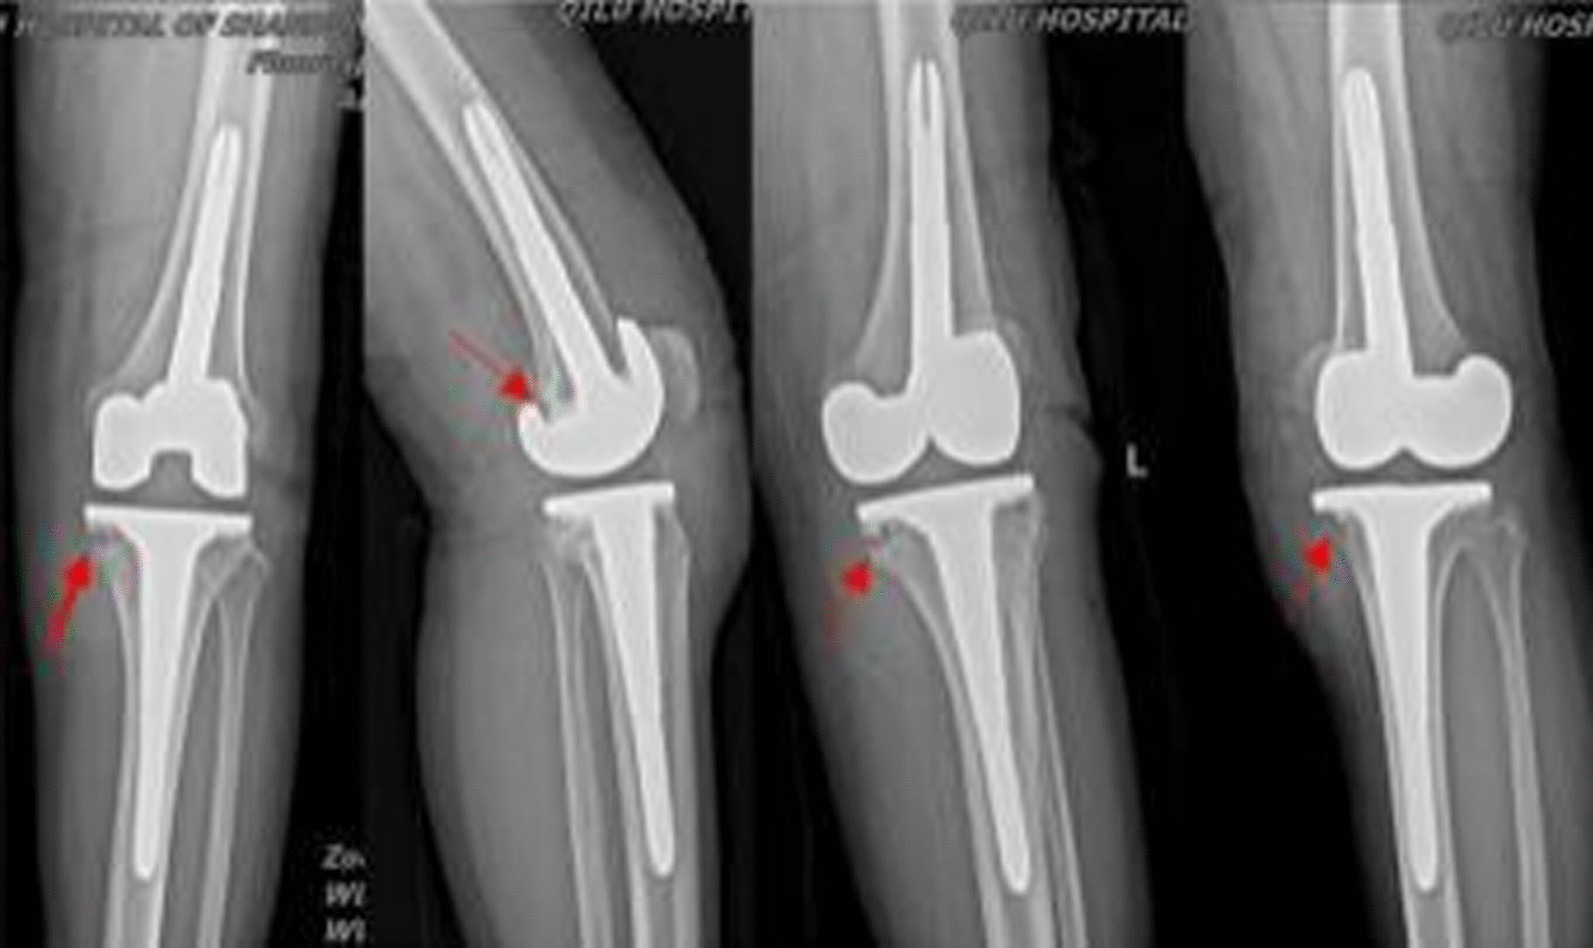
The anteroposterior, lateral, and double oblique radiographs of the left knee joint reveal translucent zones (indicated by red arrows) at the femoral posterior condyle and under the tibial prosthesis
